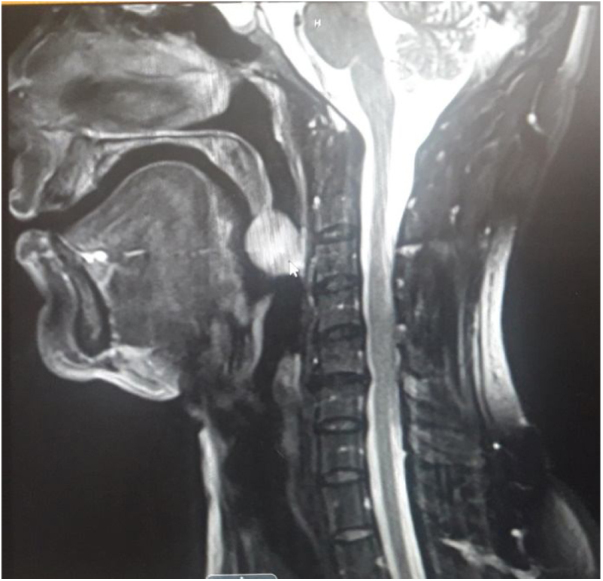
MRI showing ovoid enhancing mass lesion with well-defined margins hanging from tip of the uvula.
